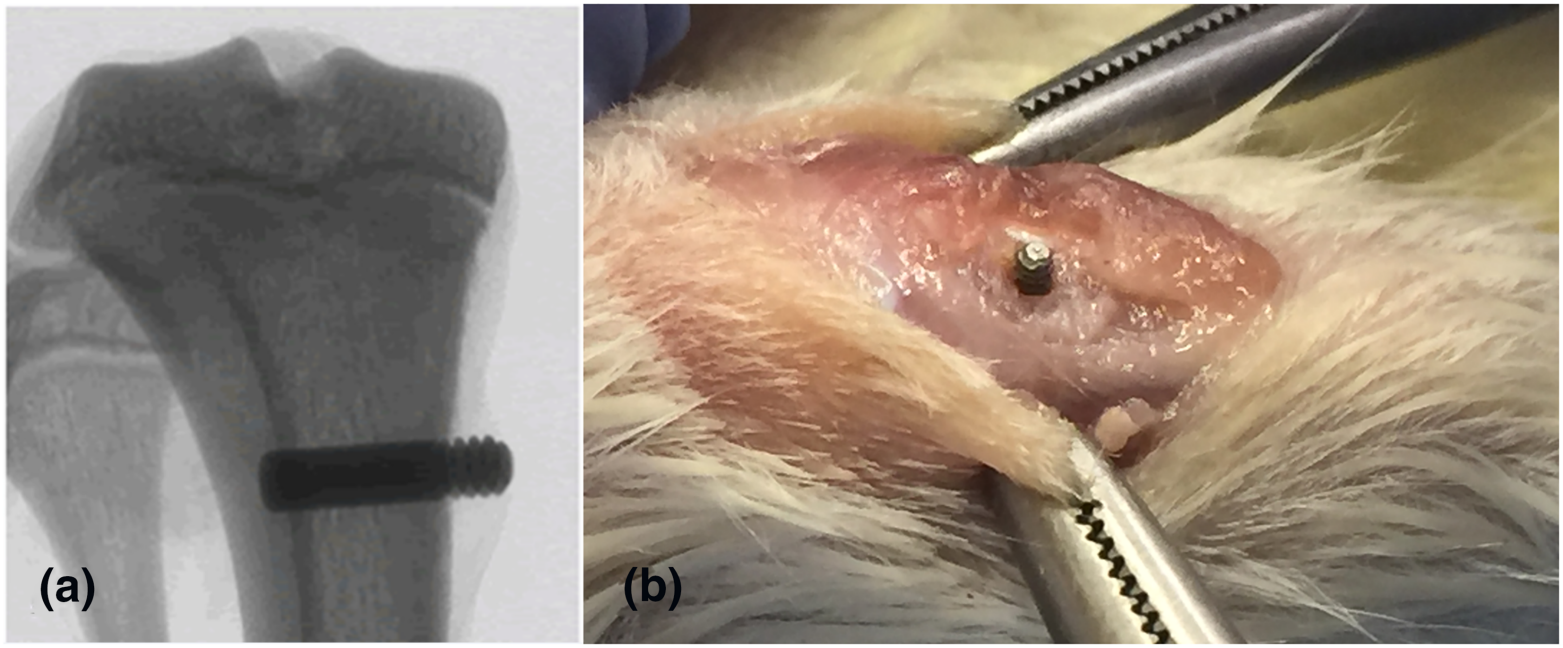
(a) Radiographic image of the implant positioned in the tibial metaphysis. (b) Photographic image of the implant positioned in the proximal tibia prior to achieving soft tissue closure
